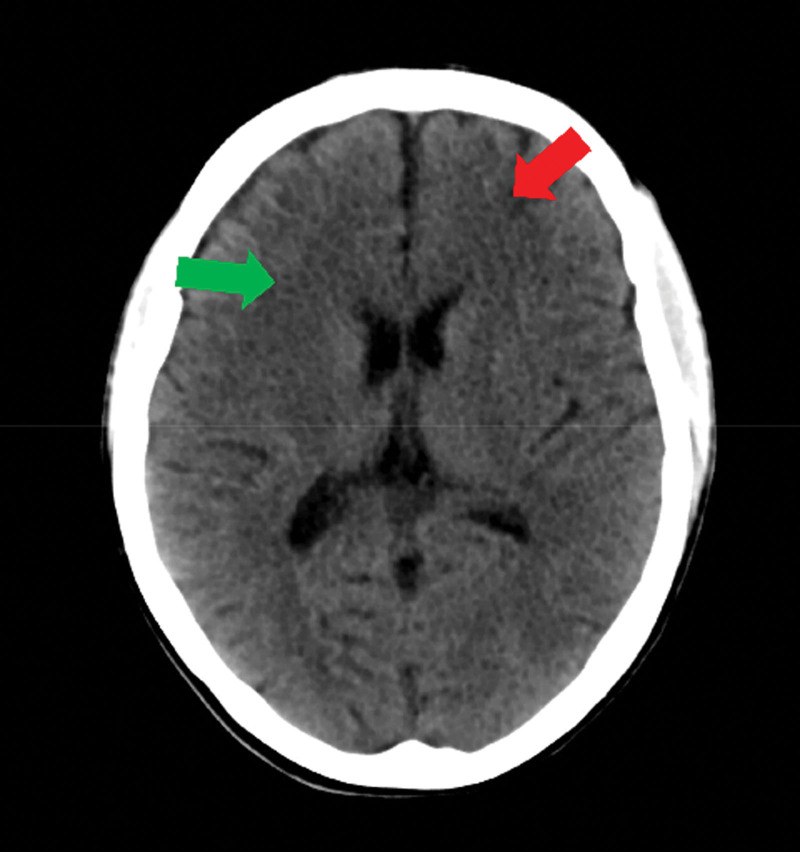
CT scan of the head showed multiple low-density foci in the bilateral frontotemporal as shown by the red and green arrows.
